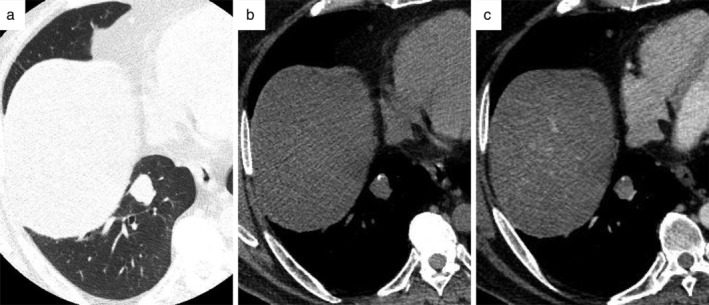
Diagnostic imaging. (a) Computed tomography (CT) with a lung window setting indicated the presence of a well‐defined solid nodule in the right lower lobe. (b) Noncontrast CT with a mediastinal window setting showed punctate calcification in the eccentric part of the nodule. (c) Contrast‐enhanced CT revealed a strong contrast enhancement of 50 Hounsfield units in the nodule.
